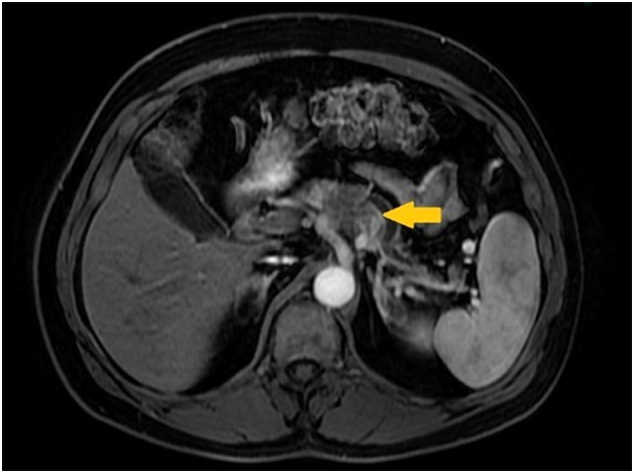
CT image before neoadjuvant chemotherapy. Nodular lesion (indicated by the yellow arrow) with hypovascular behavior measuring 3.1 × 2.8 cm in the body of the pancreas. (For interpretation of the references to colour in this figure legend, the reader is referred to the web version of this article.)
